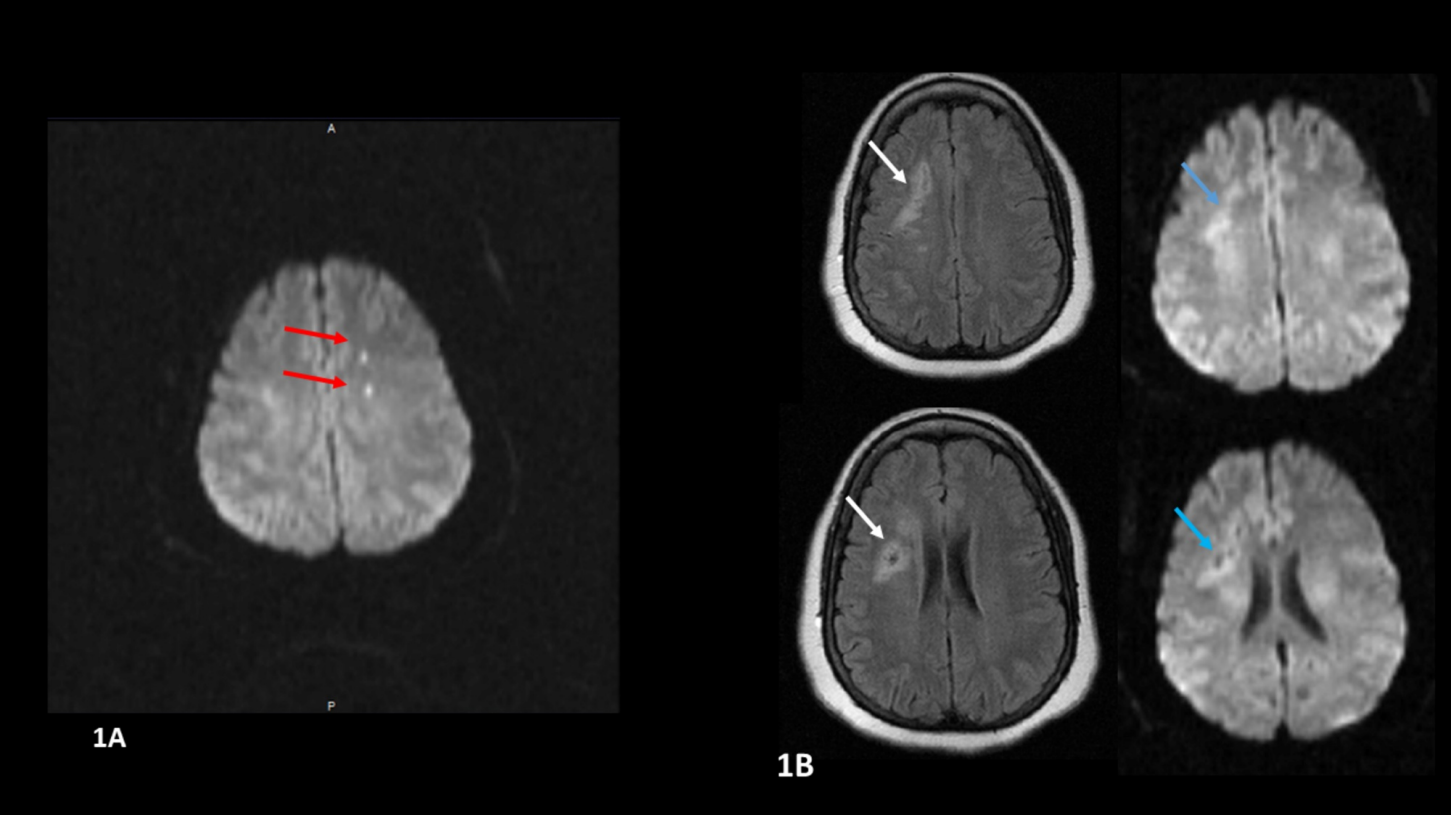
MRI of the brain MRI of the brain was done for further assessment. Figure 1A shows two foci of diffusion restriction on the diffusion-weighted sequences in the left frontal centrum semiovale consistent with acute ACA/MCA watershed infarction. Figure 1B (left: FLAIR sequences, right: diffusion-weighted sequences) shows areas of gliosis/encephalomalacia in the right frontal white matter (white arrows) with no equivalent diffusion restriction (blue arrows). Abbreviations: FLAIR, fluid attenuation inversion recovery; ACA/MCA, anterior cerebral artery/middle cerebral artery
